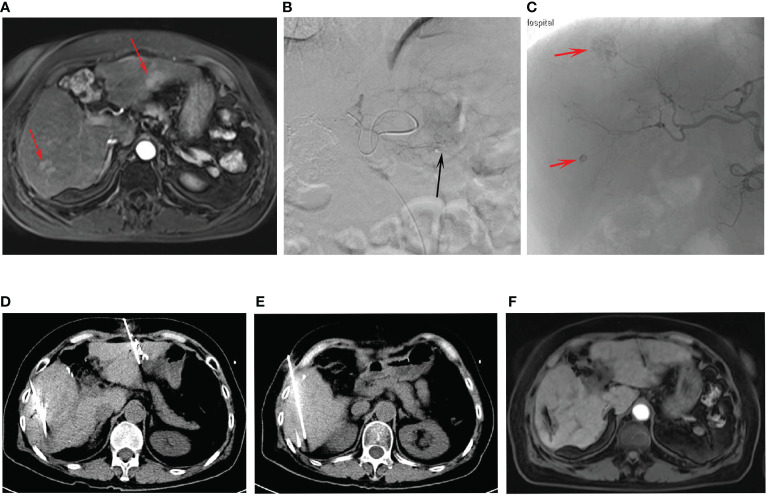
The procedures of combined treatment for multifocal HCC. (A) The preoperative contrast-enhanced MRI showed the tumors (arrows) at the left and right lobes. (B) The TACE for the left lobe tumor (arrow). (C) The TACE for the right lobe tumors (arrows). (D, E) The procedures of CT-guided ISI after TACE for the multifocal HCC. (F) The post-operative contrast-enhanced MRI indicated the treatment response was CR.
